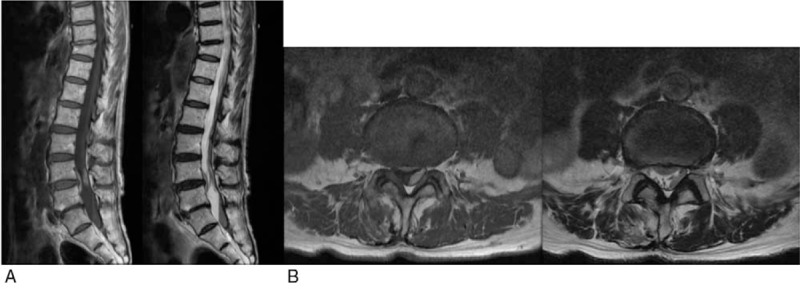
Magnetic resonance images. (A) Sagittal T1-weighted and T2-weighted images of the thoracolumbar region. (B) Axial T1-weighted and T2-weighted images at the L4/5 level.
